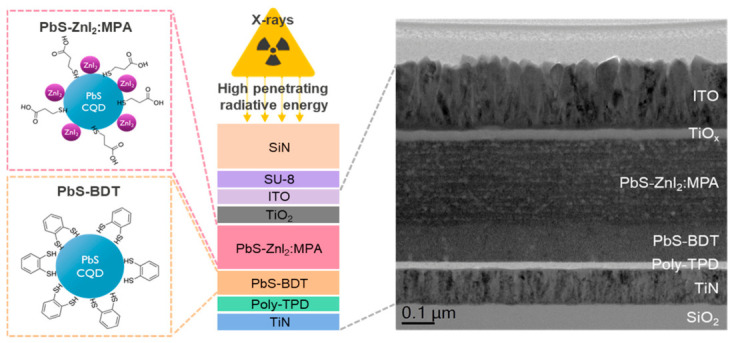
X-ray irradiation effects on CQD-TFPDs: device structure, ligand-dependent CQD behavior, radiation mechanisms, and cross-sectional HR-TEM image.
